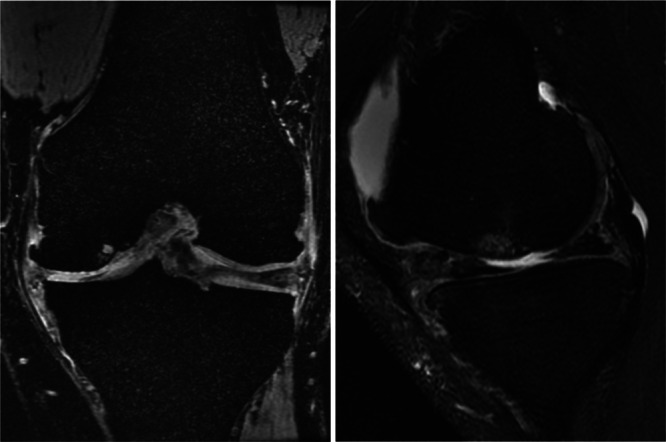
Subchondral lesions in osteoarthritic knee. In this example of magnetic resonance imaging (MRI) from a 51‐year‐old male patient with knee osteoarthritis (OA), the loss of articular cartilage is associated with subchondral damage, bone marrow oedema‐like lesions, and bone cysts.
